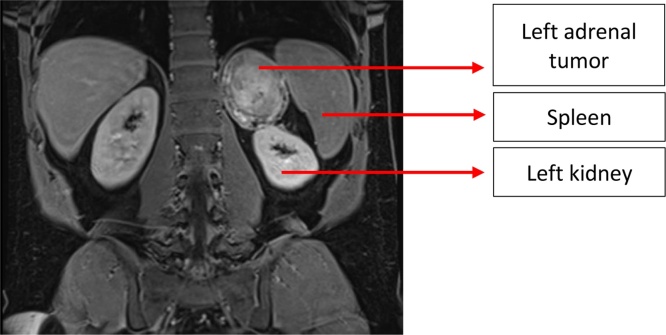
MRI showing the large tumor adjacent (in contact) with the left kidney.
